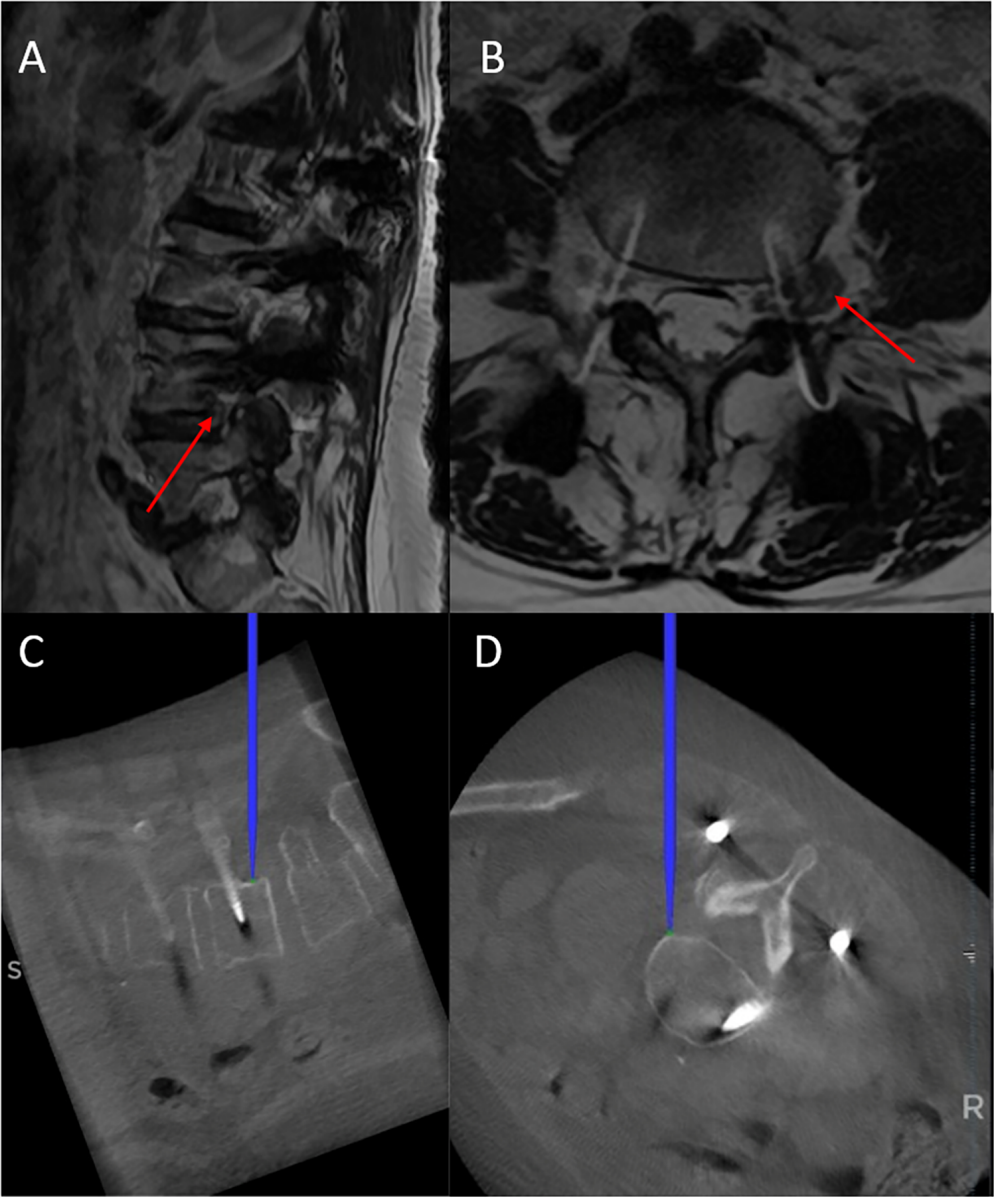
Upper: pre-operative T2-WI (A) sagittal and (B) axial MRI. Disc herniation at left L4–L5 foraminal-extraforaminal localization just beneath pedicle screw was evident (red arrow). Lower: (C) sagittal and (D) axial intraoperative CT-navigation showing the tip of the navigation probe positioned at the level of extruded fragment, allowing its rapid identification and removal.
